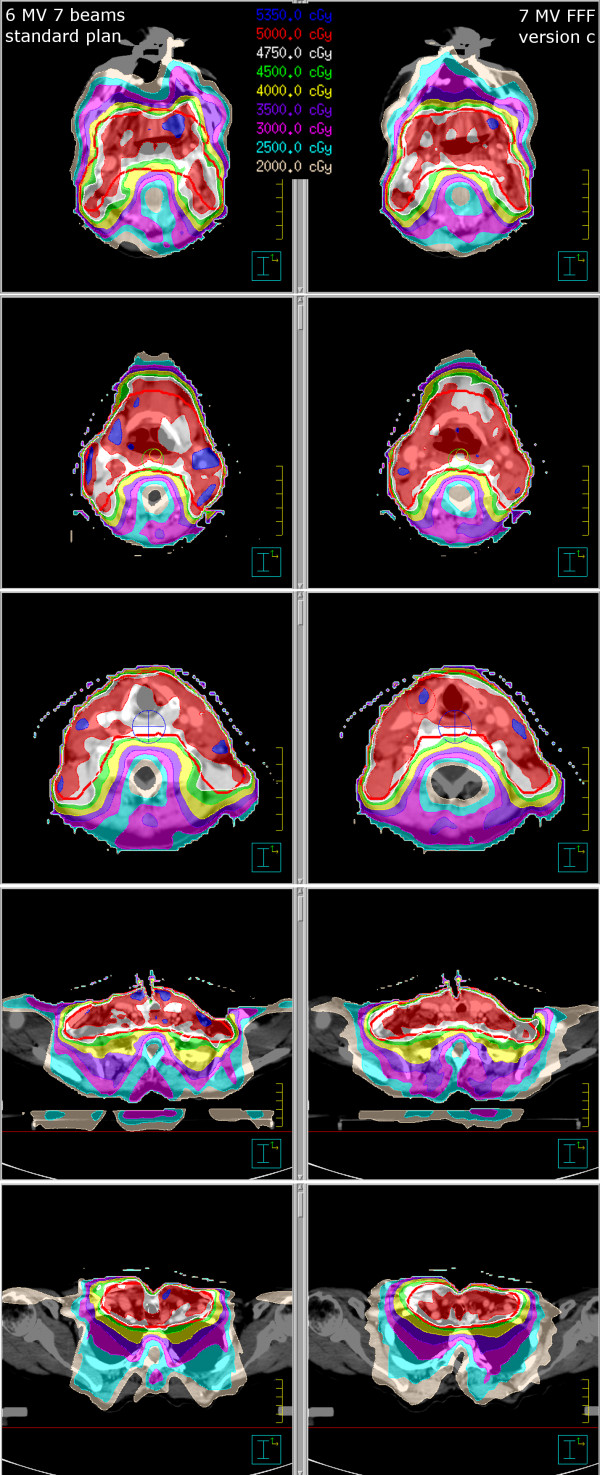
Example dose distributions of head-and-neck treatment plan. The left panel shows our standard IMRT plan (also displayed in Figure 5 as thick lines in the DVH), the right panel shows version c converted plan (FFF 7 MV), both in different CT slices.
